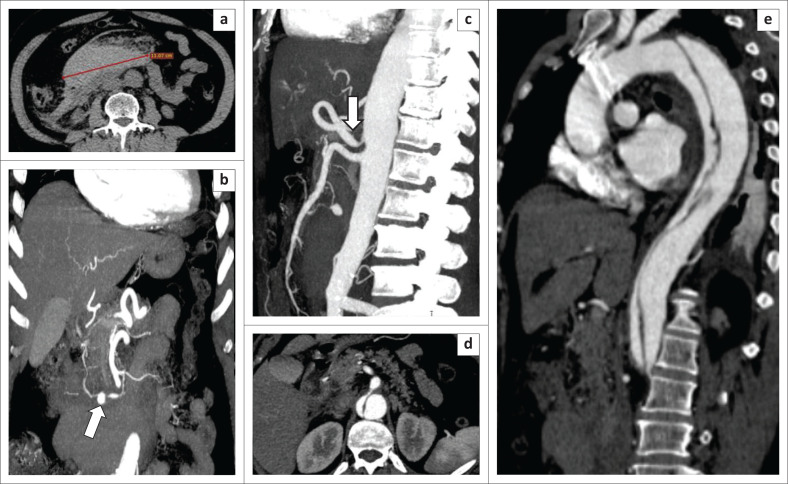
A 44-year-old woman admitted with haematemesis and melena. Non-enhanced computed tomography in the axial plane (a) showing a homogeneous hyperdense haematoma of approximately 11 cm. Arterial phase in coronal (b), sagittal (c), axial (d) and oblique plan (e) revealed a small aneurysm (arrow, b) originating from the inferior pancreatic-duodenal artery (IPDA) (arrow, b); Moderate coeliac artery compression by the median arcuate ligament (arrow, c) is identified. There is a Standford B aortic dissection (e) where the coeliac artery originantes from the false lumen (d).
